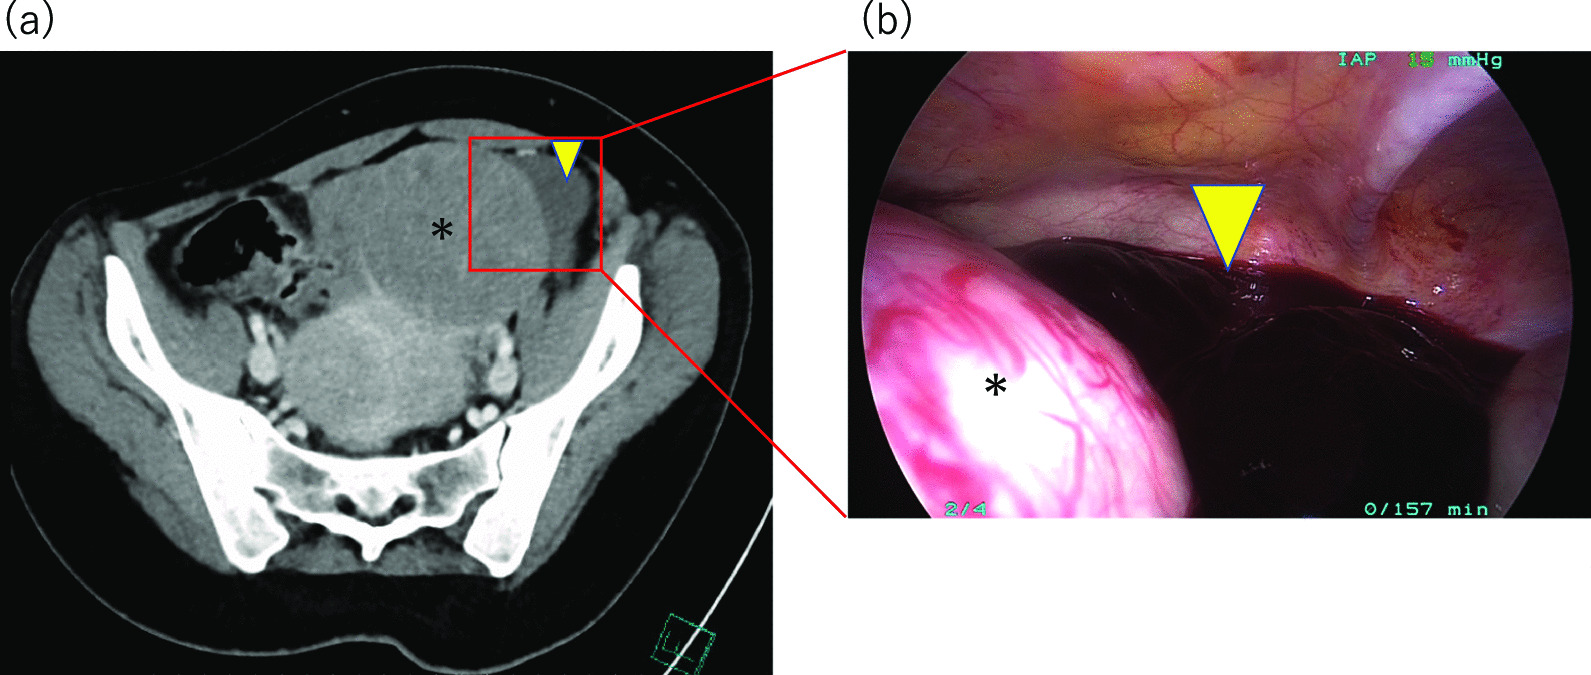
Preoperative computed tomography and intra-operative laparoscopy images identifying the hemoperitoneum. a Abdominal contrast-enhanced computed tomography showed a 7.9-cm fibroid (asterisk) and peritoneal fluid with an imaging density suggestive of blood (yellow arrowhead). b Exploratory laparoscopy identified the hemoperitoneum consistent with computed tomography (yellow arrowhead)
